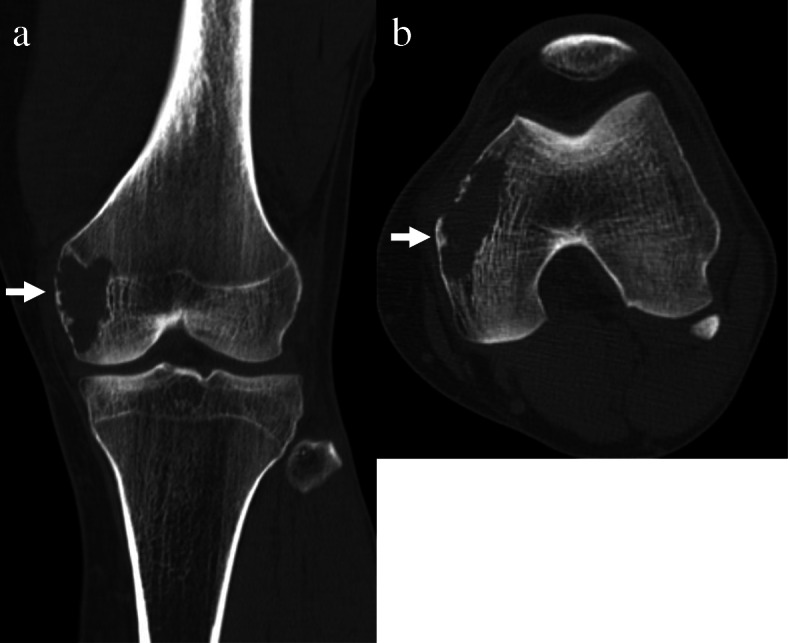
Computed tomography scans show a lytic lesion with partial cortical destruction in the distal femur. Lytic lesions (arrows) with partial cortical destruction are shown in (a) 3D coronal view and (b) Axial view
